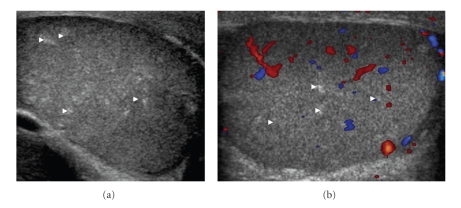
26-year-old patient presented with painless swelling in the right scrotum. (a) Grey Scale Ultrasound of Scrotum showing multiple small ill defined and randomly distributed echogenic foci within the testicular substances (white arrowheads). (b) Colour Doppler showing normal vascularity of testis. However, the lesions are typically avascular.
